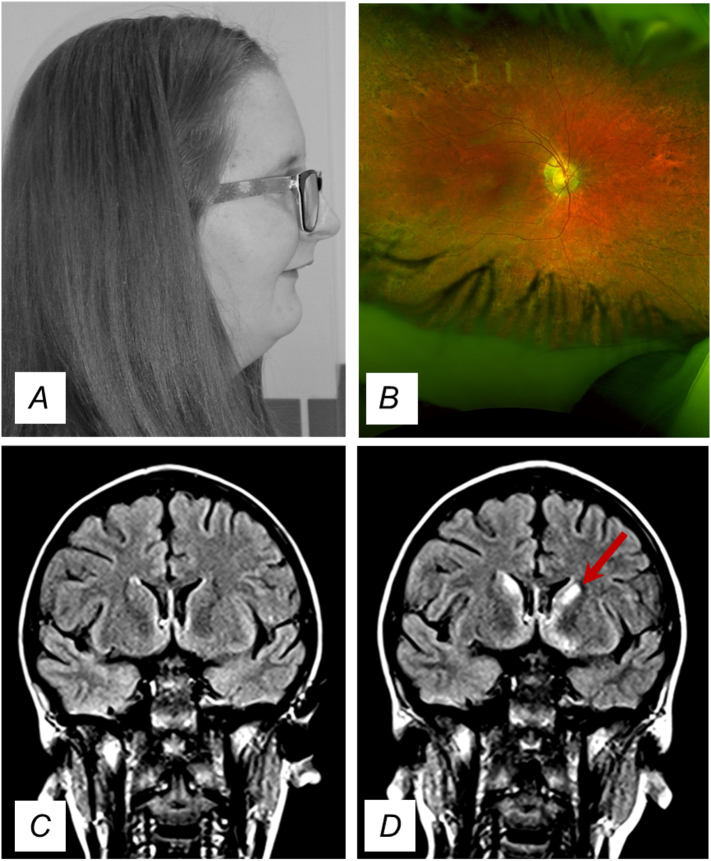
Clinical features. (A) Clinical photography of the patient (aged 16 years) showing an underdeveloped lower jaw. (B) Retinal photography revealed pigmentary changes on her retina. (C) MRI T2 FLAIR coronal view performed at the age of 12 years showed normal T2 signal in the caudate nucleus. (D) Similar view performed at the age of 13 years showed high T2 signal in the caudate nucleus bilaterally; the red arrow indicates this abnormality on the left. This MRI signal change is likely to have developed during the 1 year interval between the two scans and coincides with a deterioration in her gait. (For interpretation of the references to colour in this figure legend, the reader is referred to the web version of this article.)
